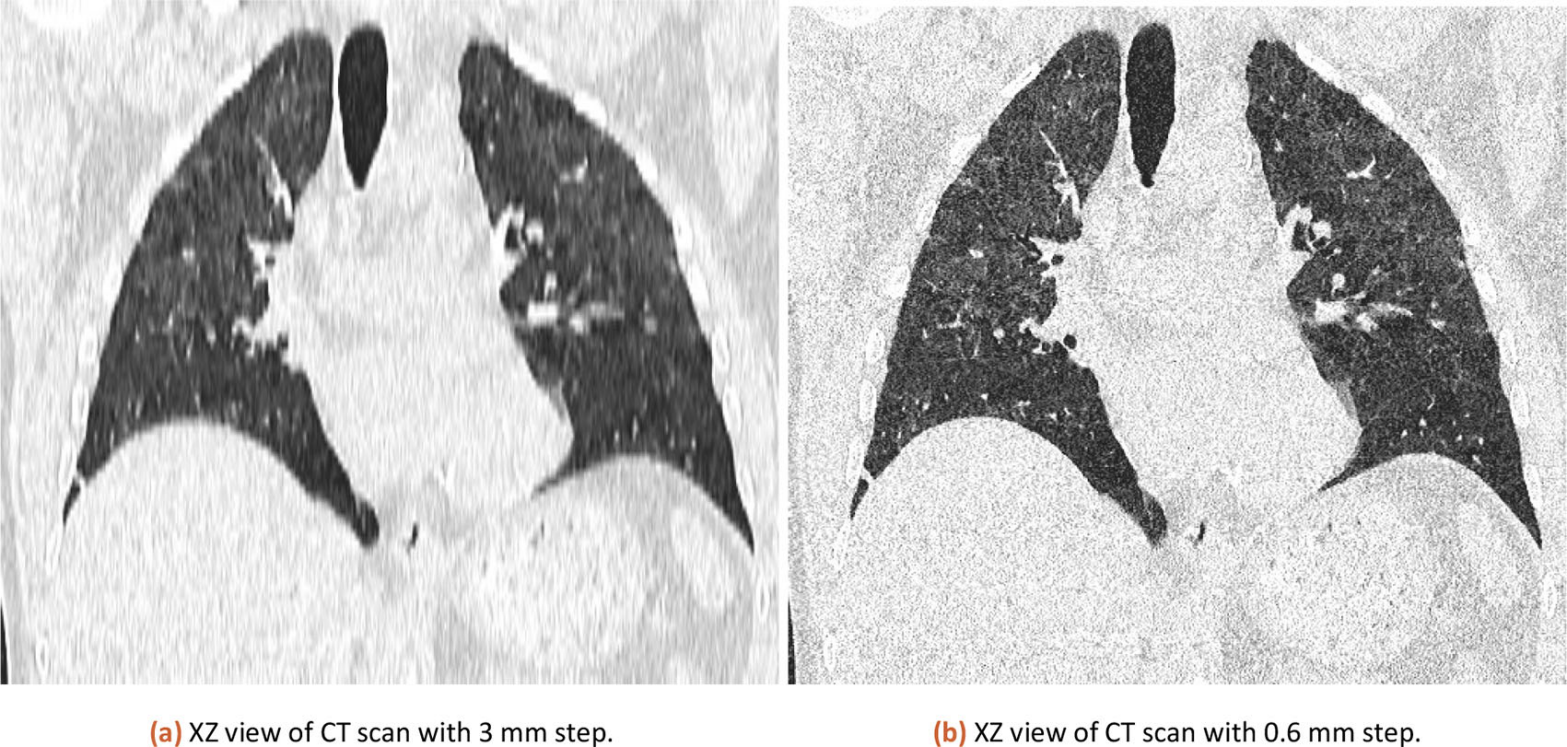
XZ view comparison of 3 mm and 0.6 mm CT.
